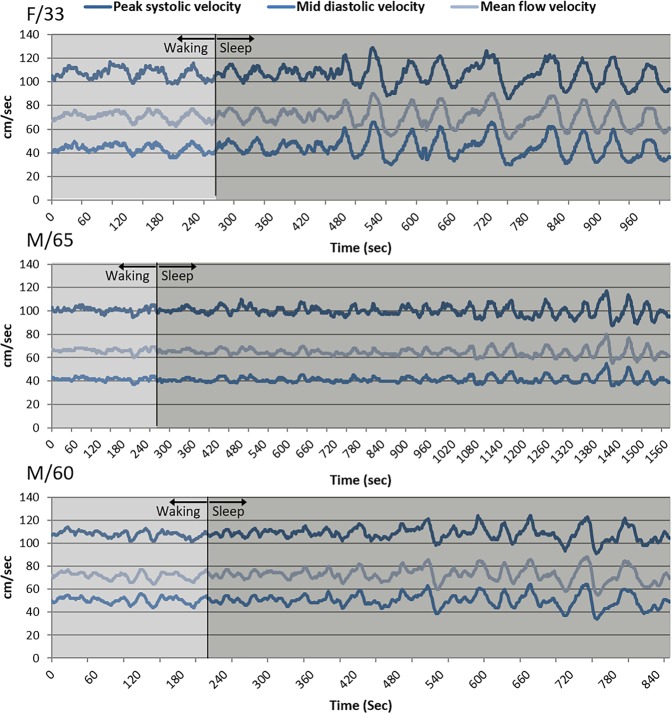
Changes in the mean flow velocity in sleep monitoring: Representative cases. Transcranial Doppler monitoring during short-term non-REM sleep in a 33-year-old woman (upper panel), a 65-year-old man (middle panel), and a 60-year-old man (lower panel) showed a marked amplification of the periodic patterns in the peak systolic velocity, mid-diastolic velocity, and mean flow velocity graphs with a period of approximately 50 s, which appeared within a few minutes after the onset of sleep.
